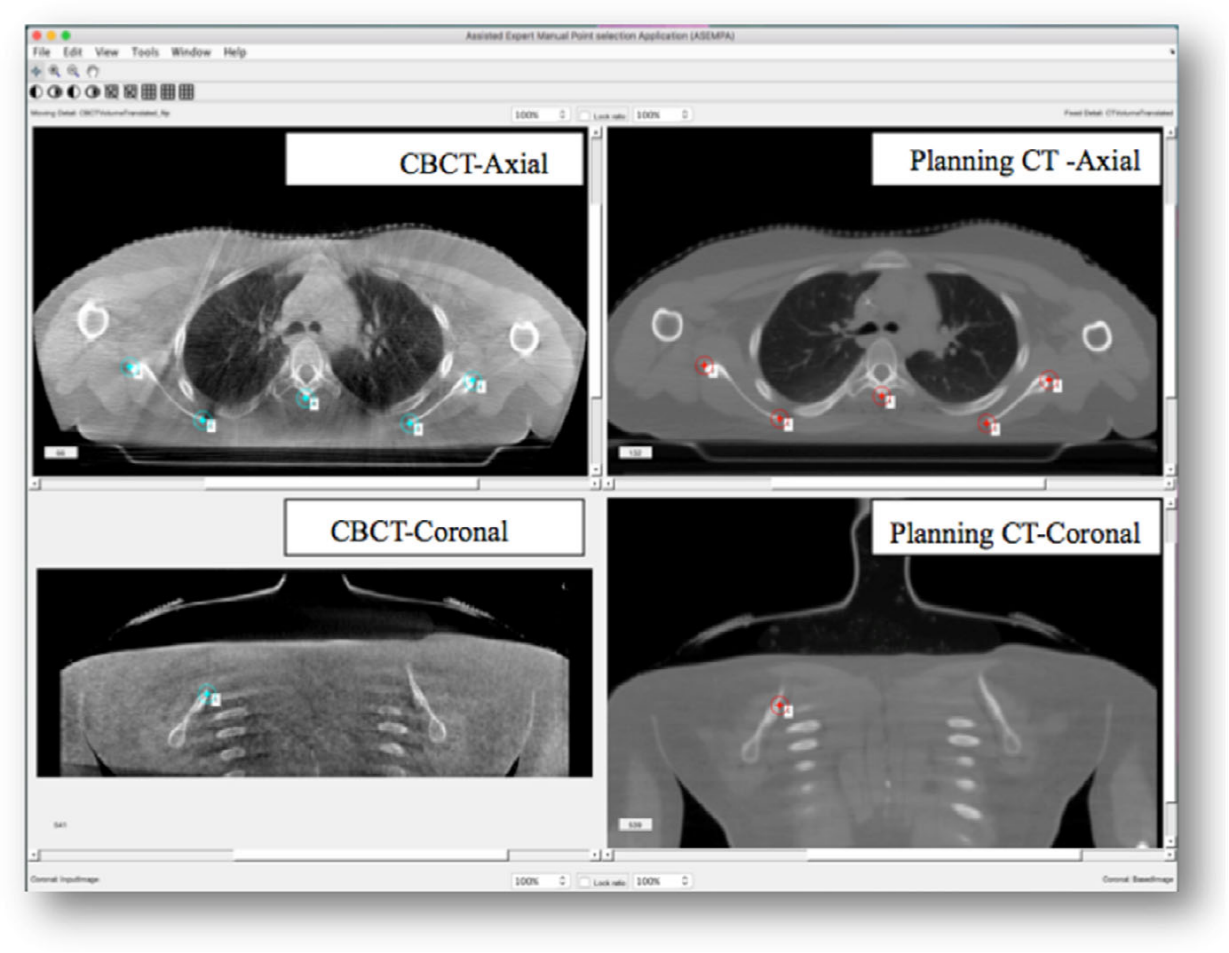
Assisted expert manual point selection application interface showing sample data. The Image on the left panel (top and bottom) shows cone beam computed tomography (CBCT) of the target image and image on the right panel (top and bottom) shows the planning CT image, which is the reference image.
